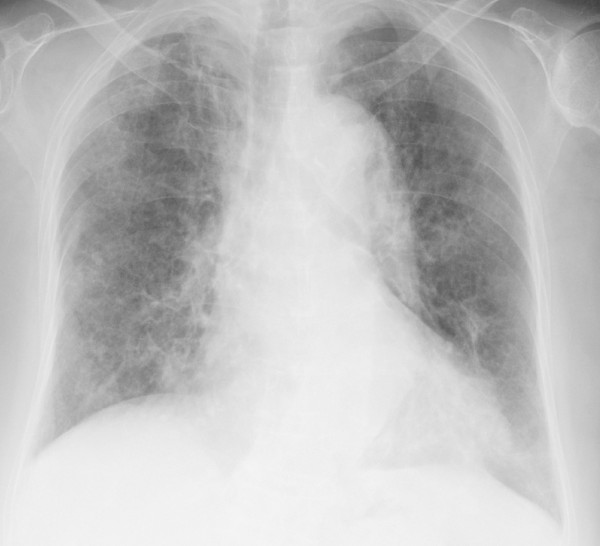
Chest X-ray obtained after the diagnosis of pneumocystis pneumonia showed areas of ground-glass opacity bilaterally in almost all lung fields.
